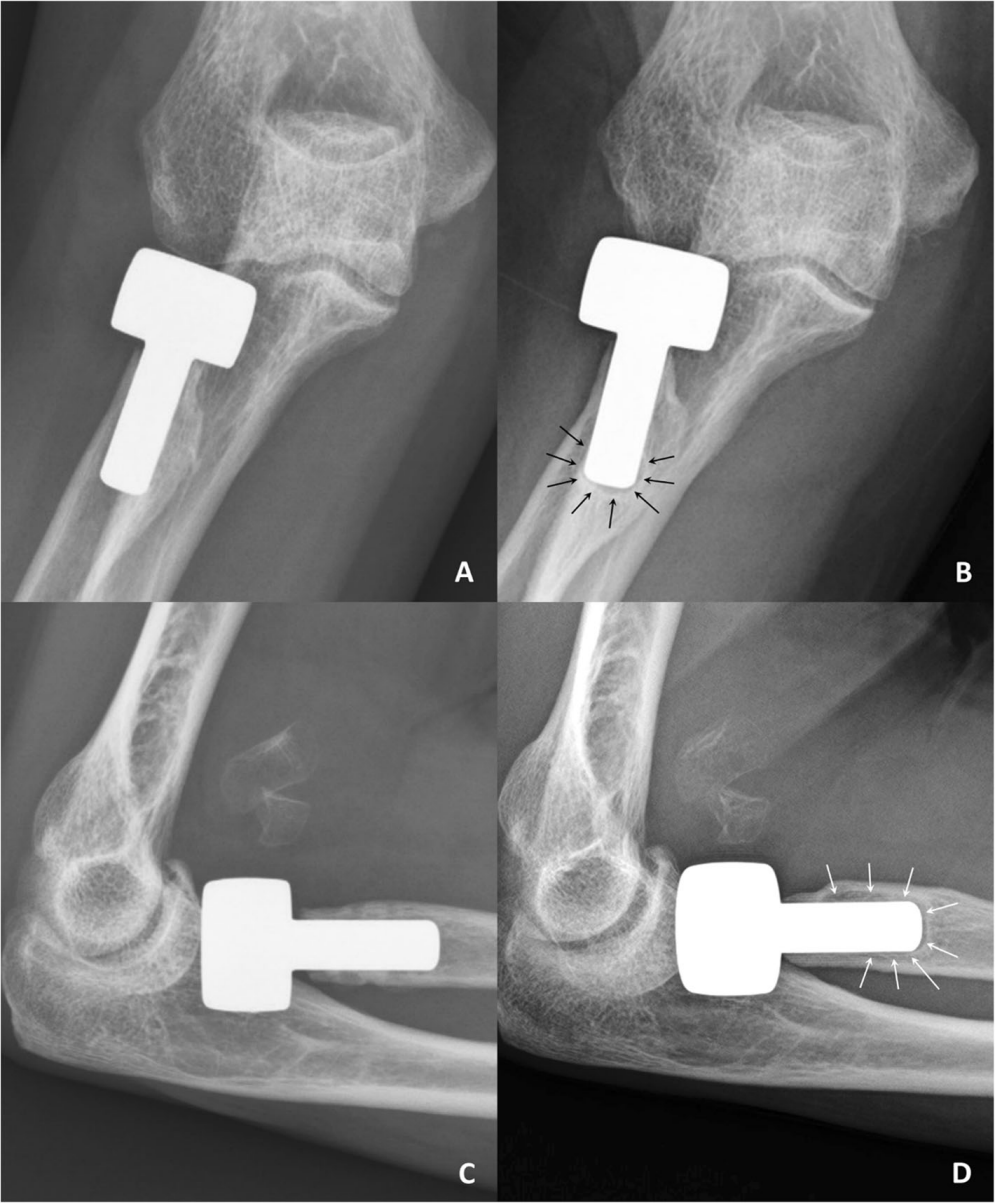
Right elbow radiographs from a 54 year-old female patient after radial head arthroplasty. a Anteroposterior view at 3 months. b Anteroposterior at 11 years showing periprosthetic osteolysis (black arrows). c Lateral view at 3 months. d Lateral view at 11 years showing periprosthetic osteolysis (white arrows)
